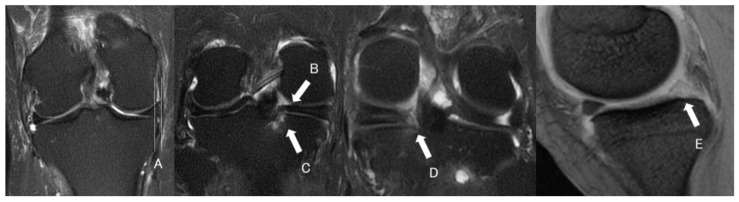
(A) Medial meniscus extrusion (MME) was measured at the center of the tibial plateau. Two vertical lines were drawn—one tangential to the medial edge of the tibial plateau and another parallel line along the outer edge of the medial meniscus—and the distance between the two lines was defined as MME. (B) A lateral view of a giraffe neck–like shape of the medial meniscus posterior segment on coronal MRI was defined as the giraffe neck sign. (C) Radial separation of the posterior root fibers resembling divergent roots was defined as the spreading roots sign. (D) An abrupt discontinuity of the posterior root contour was defined as the truncation sign. (E) Loss of the normal intrameniscal signal, appearing as a homogeneously white structure, was defined as the white meniscus sign.
